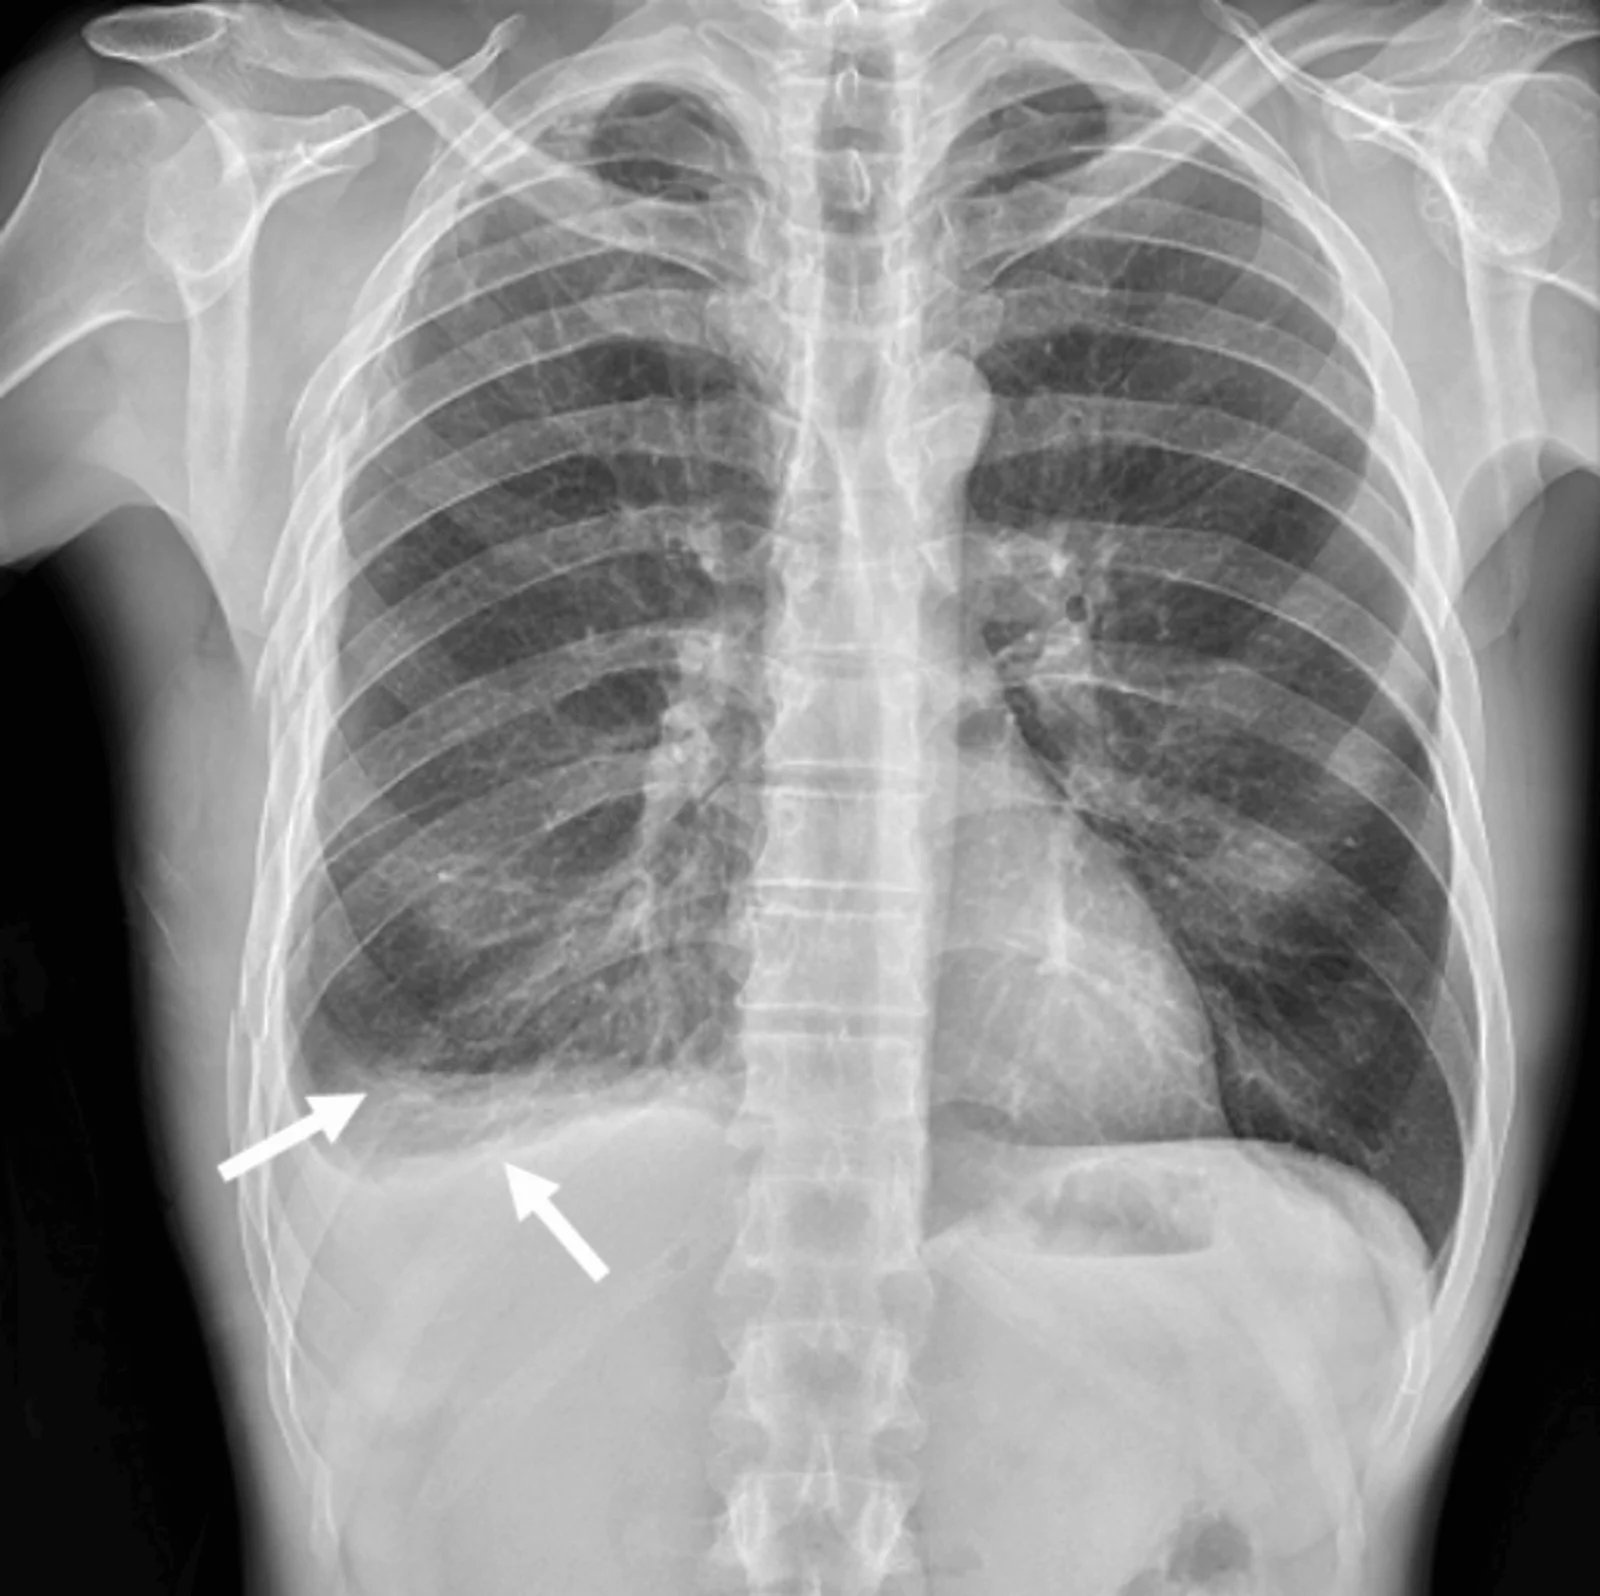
Anteroposterior chest radiograph obtained post-chest tube removal on day six of admission. An upright frontal chest radiograph obtained immediately following the removal of the right-sided chest tube. Compared to the previous radiograph, the lateral white arrow highlights blunting of the right costophrenic angle and localized pleural thickening, while the medial white arrow points to a small residual subpulmonic fluid collection. The lungs are otherwise well-expanded with no significant residual lateral or apical pneumothorax.
